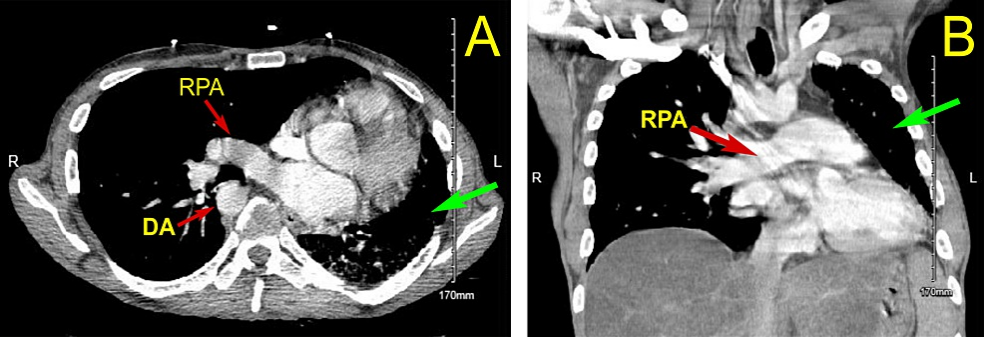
CT thorax with IV contrast, axial and coronal images CT thorax with IV contrast axial image (A), and coronal image (B) demonstrate an absent left pulmonary artery, enlarged right pulmonary artery, and right-sided aortic arch. In addition, the left lung exhibited marked hypoplasia (green arrows). RPA - right pulmonary artery; DA - descending aorta
